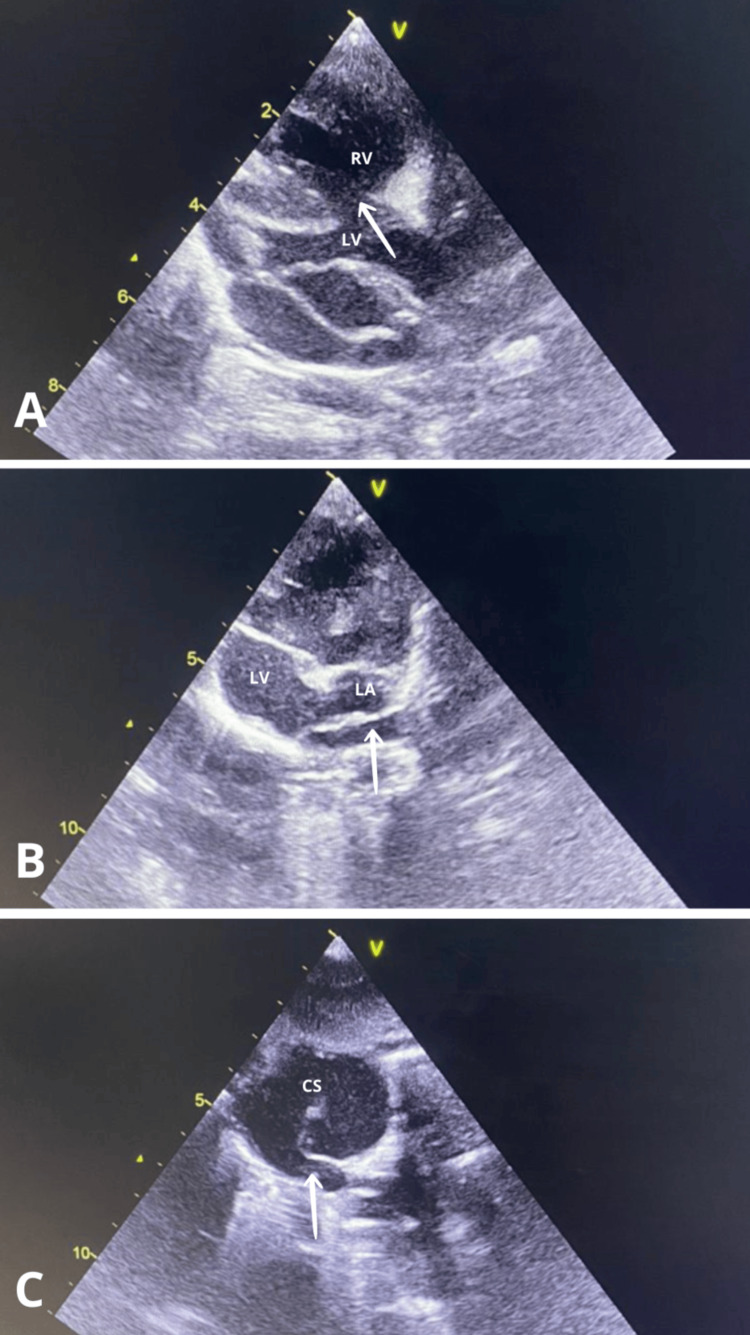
Echocardiography two-dimensional mode: (A) Ventricular septal defect (arrow); (B) The retroauricular collector where the pulmonary veins drain (arrow); (C) The collector of the pulmonary veins (arrow) which drains into the coronary sinus and then into the right atrium. LV: left ventricular; RV: right ventricular; LA: left atrium; CS: coronary sinus
